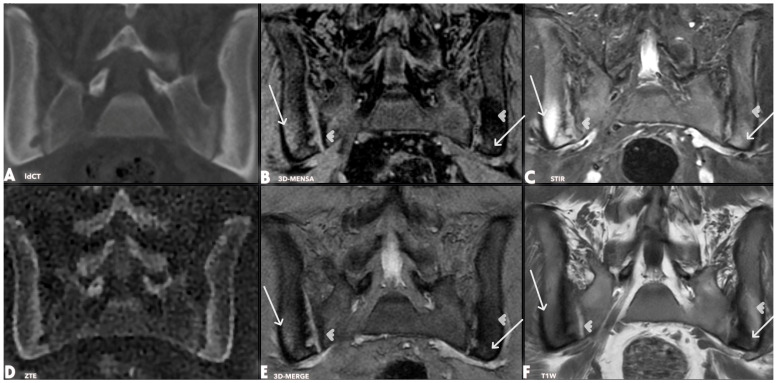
Coronal oblique images of a 17-year-old male patient were acquired from the same slice using low-dose CT (A), 3D-MENSA (B), STIR (C), ZTE (D), 3D-MERGE (E), and T1W (F) sequences. White arrowheads indicate fat metaplasia—hypointense on 3D-MENSA (B), hyperintense on T1W (F), and faintly visible on STIR (C) and 3D-MERGE (E); not detected on ZTE (D) or low-dose CT (A). White arrows show bone marrow edema, which appears hyperintense on 3D-MENSA (B) and STIR (C), slightly hyperintense on 3D-MERGE (E), and not visible on low-dose CT (A) or ZTE (D).
